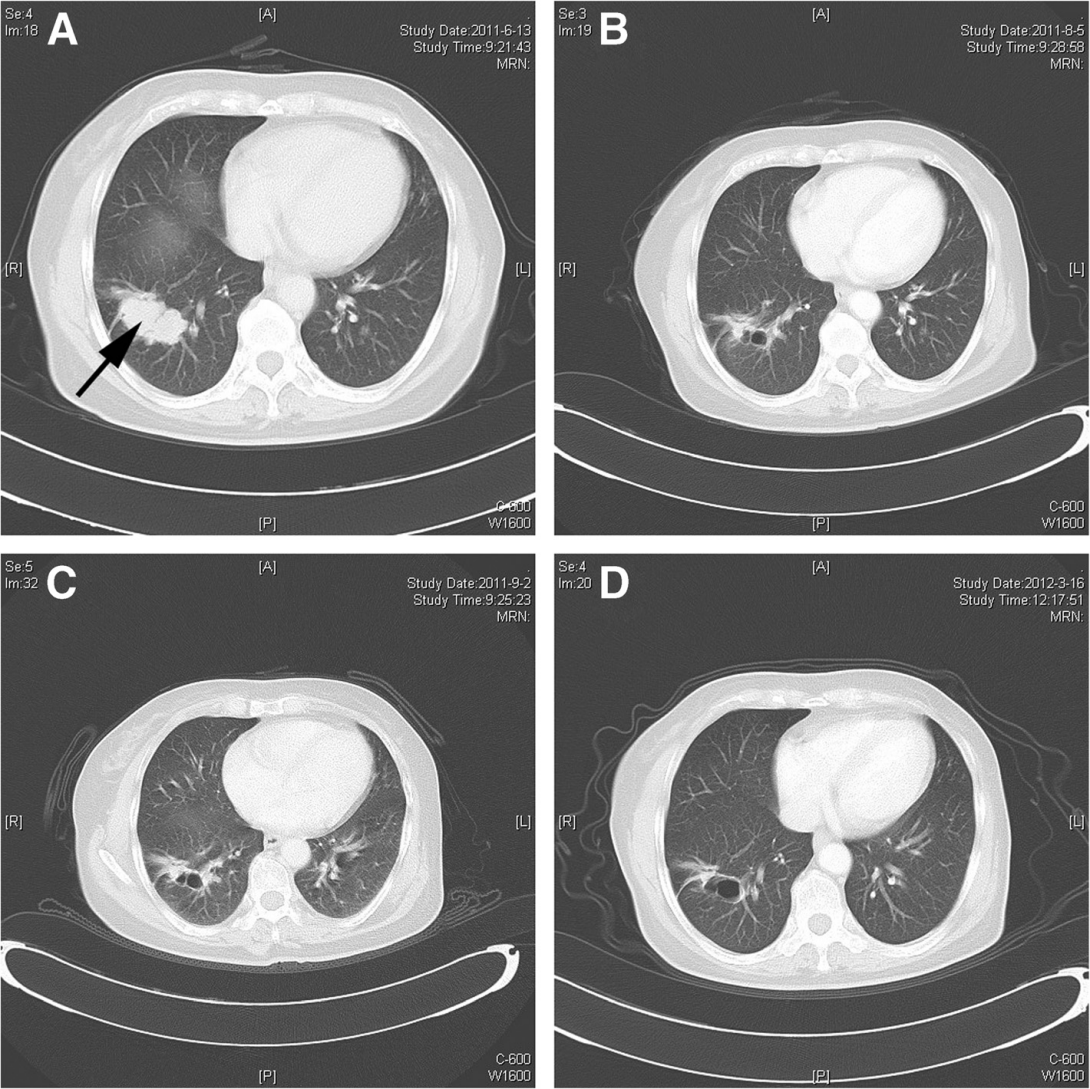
A 67-year old female patient diagnosed with MBC who had experienced treatment failure after 2 chemotheraphy and 2 hormonal regimens, but did not receive trastuzumab. She had confirmed PR at the 1500 mg BID dose level of AST1306, but withdrew from the study after 9 months due to continuous grade 2 diarrhea. A) CT imaging of the metastatic lesion prior to starting therapy, and B) shows the response to AST1306 two cycles after starting therapy. C and D shows continued response three and ten cycles after starting therapy.
